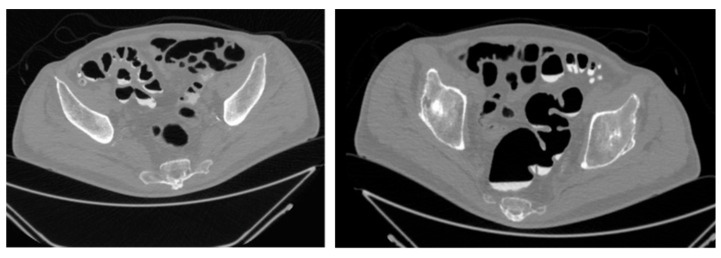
CT—Virtual Colonoscopy: Abdominal CT scan (virtual colonoscopy reconstruction) showing diffuse diverticulosis of the sigmoid colon with associated wall thickening. A perivisceral inflammatory reaction is also evident around the affected area.
